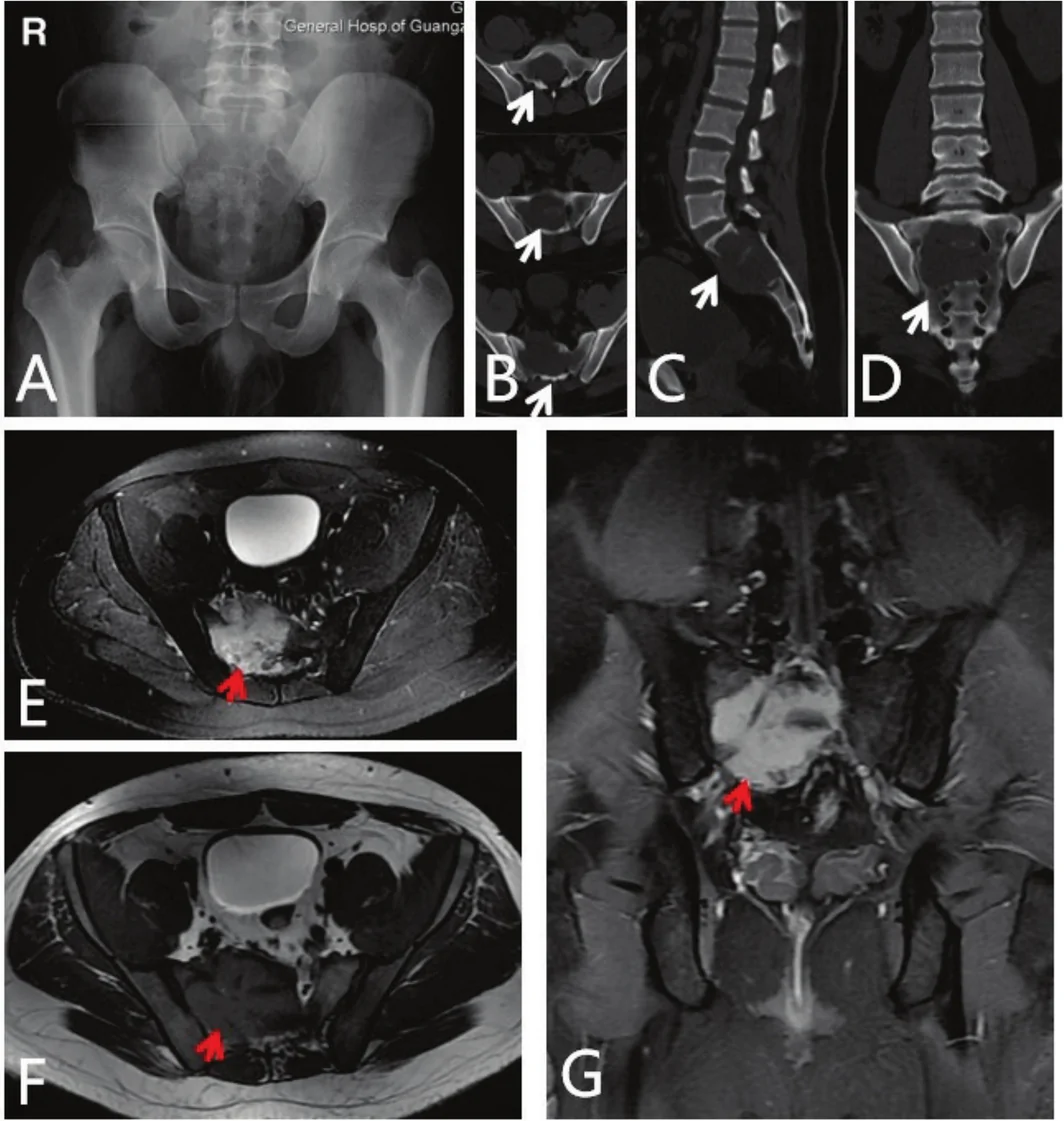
A 24‐year‐old male patient with lumbosacral pain for 2 months and dysuria for 1 month. (A) Preoperative anteroposterior radiograph of the pelvis and (B–D) preoperative CT scans demonstrating bone destruction involving S1, S2, S3 and the right‐sided region, with disappearance of the S1 and S2 anterior sacral foramina. (E–G) Preoperative MRI showing tumor infiltration of S1‐S3, extending rightward to the sacral margin and downward to mid‐S3.
